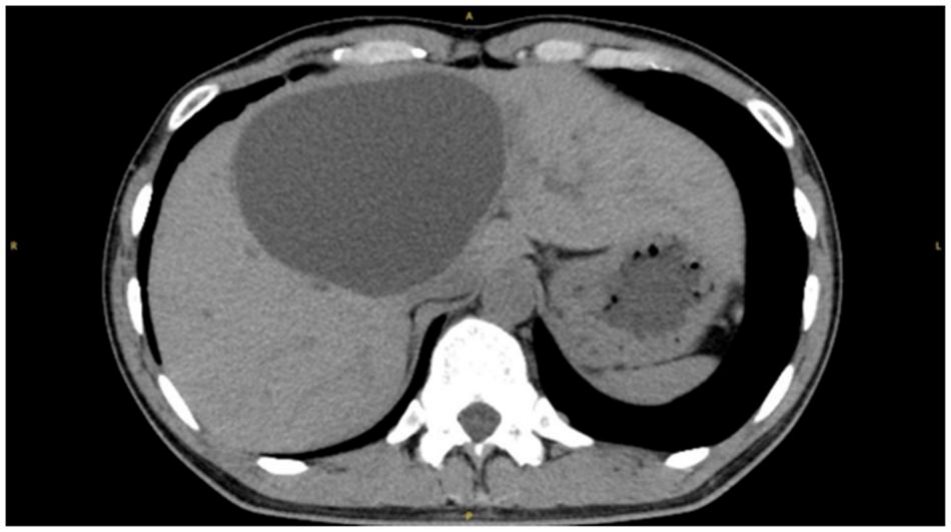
Non-enhanced computed tomography of the upper abdomen shows a large cyst in hepatic S4.
